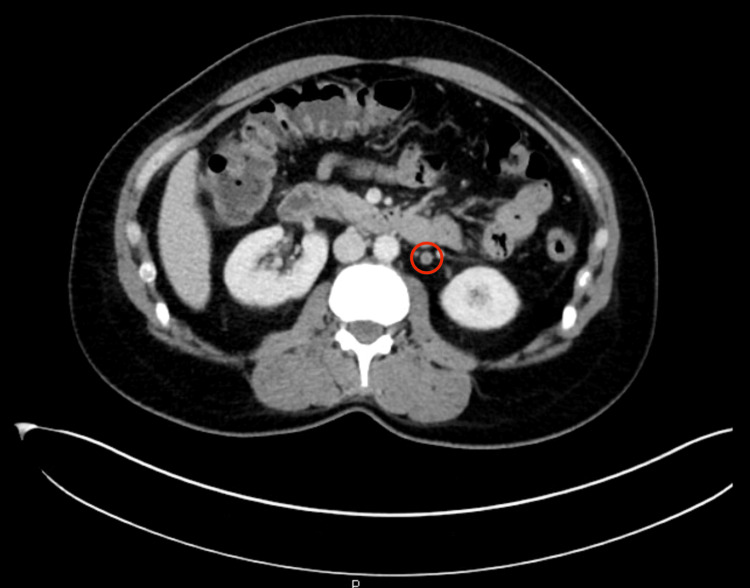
Transverse CT of the abdomen and pelvis demonstrating left gonadal vein filling defect
